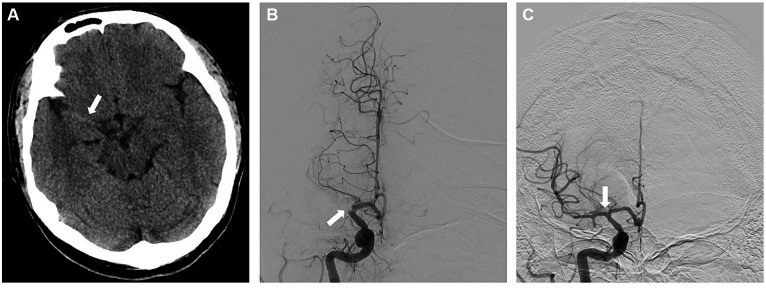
(A) Non-contrast CT showing the right-sided hyperdense vessel sign in the MCA. (B) AP view of the right ICA injection on the digital subtraction angiogram (DSA), showing the proximal right M1 cutoff. (C) AP view of the right ICA injection following successful reperfusion, early arterial phase.
